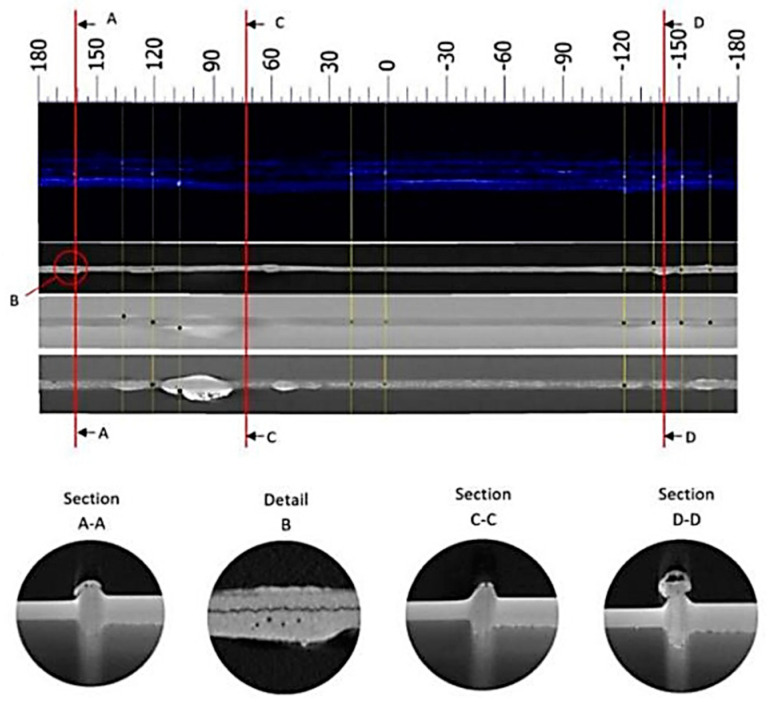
Comparison of B-scan and CT results in the cross sections of the shaft A–A, C–C, D–D and detail B [55].
